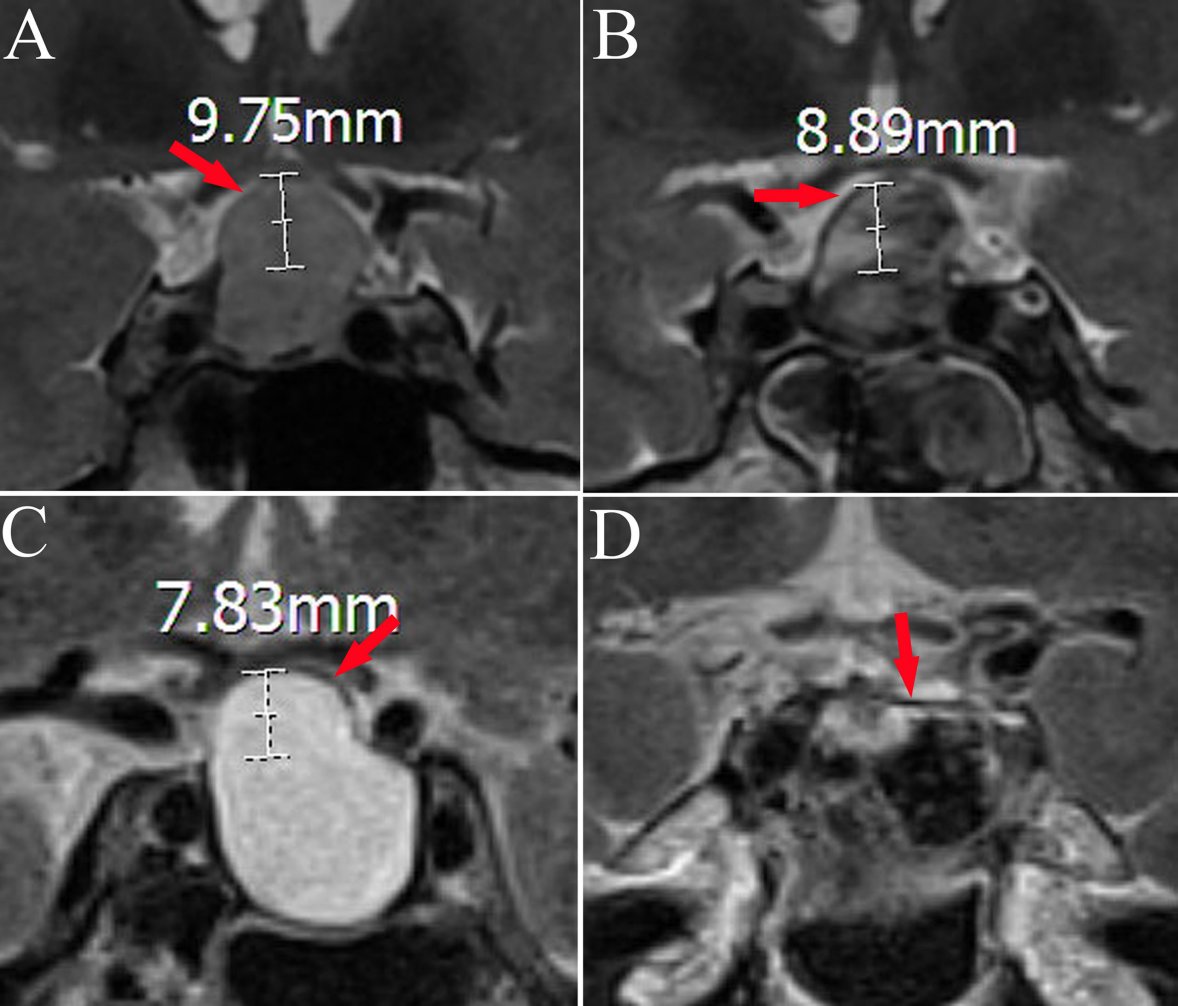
Magnetic resonance changes in the diaphragma sellae before and after transsphenoidal surgery in two cases. Panels A, B and panels C, D are from the same patient; diaphragma sellae (red arrow) (A). Hypointense shadow of diaphragma sellae in preoperative T2-weighted imaging (diaphragma sellae height 9.75 mm). (B) The diaphragma sellae in postoperative T2-weighted imaging (diaphragma sellae height 8.89 mm). (C) Hypointense shadow of diaphragma sellae in preoperative T2-weighted imaging (diaphragma sellae height 7.83 mm). (D) The diaphragma sellae in postoperative T2-weighted imaging (diaphragma sellae height 0 mm).
